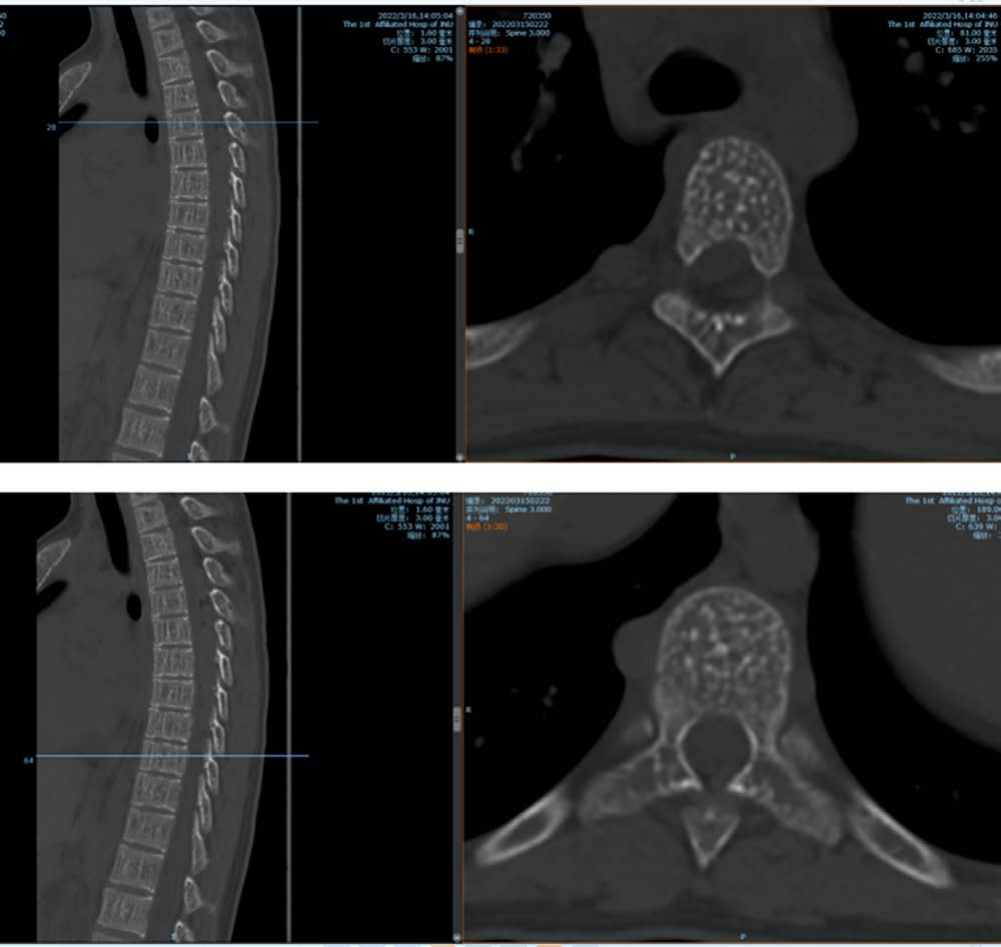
Computed tomography (CT) soft tissue window.
